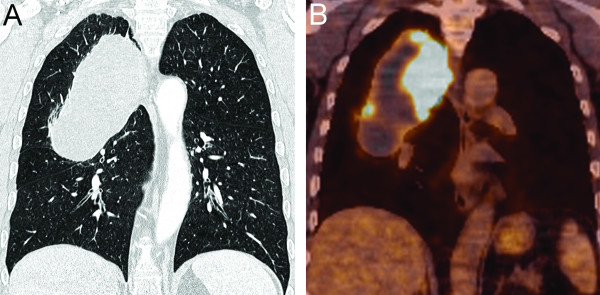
Radiographic findings of the blastomatoid pulmonary carcinosarcoma. Preoperative computed tomography (CT) detected a sharply marginated tumour in the upper right hemithorax of up to 12.9 cm size (A, coronal view). The tumour extended to the right hilus, pleura, and the spinal column. Positron emission tomography with integrated computed tomography (FDG-PET/CT, Philips Gemini, PET-acquisition 90 minutes after intravenous injection of 198 megabecquerel 2-(^18^F)-fluoro-2-deoxy-D-glucose) revealed marked FDG-glucose uptake in the pulmonary mass, especially median, lateral and caudal, with a maximum standardized uptake value (SUV_max_) of 14.1 (B, fusion PET/CT, coronal view).
